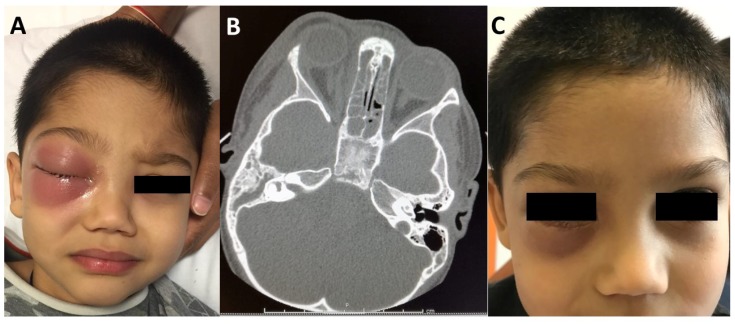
(A) A 3-year-old patient with a right sub-periosteal abscess extending to the lower lid and causing proptosis. (B) Computed tomography (CT) findings demonstrating bilateral ethmoiditis and sphenoidal sinusitis—the white arrow indicates the presence of right ethmoiditis, while the black arrow indicates a sub-periosteal abscess of the intra-orbital portion of the *lamina papyracea*. (C) The same patient 10 days after combined endoscopic surgery and external lower lid incision.
